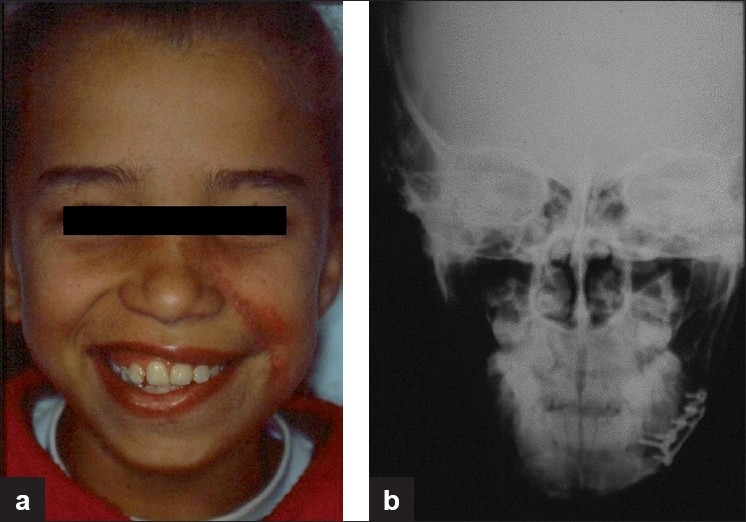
Clinical (a) and radiographic (b) aspects after 7 months
